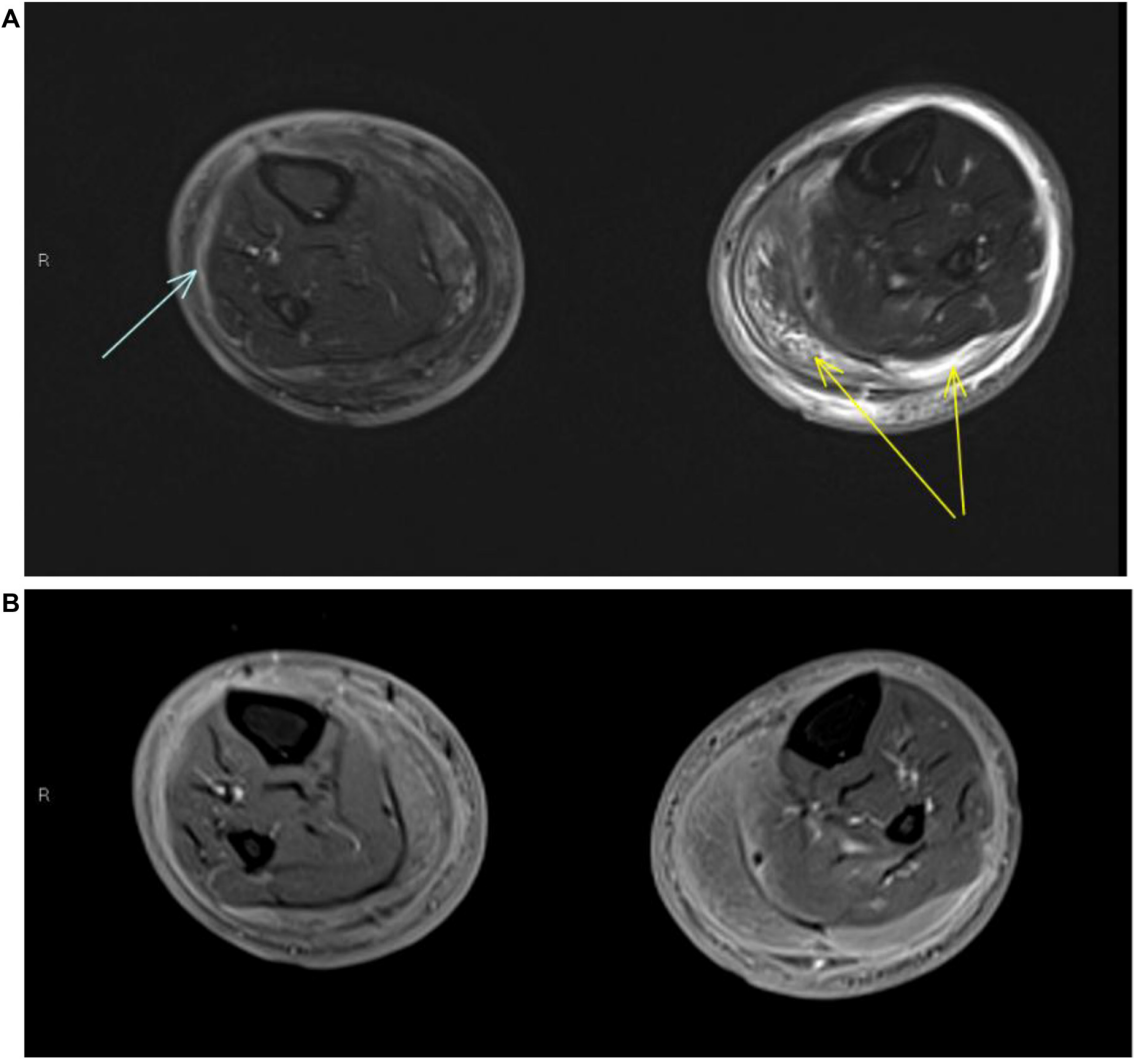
MRI imaging of a 29-year-old male patient with IgG4-related lower limb fasciitis. Axial STIR MRI (A) and postcontrast (B) images demonstrate circumferential thickening of the deep fascia of both lower legs, in keeping with recurrent fasciitis. Superficial circumferential oedema is seen on the right side while a deeper circumferential layer of subcutaneous oedema is seen on the left. Mild oedema deep to the deep fascia on the right side is suggestive of more acute inflammation (blue arrow). The muscles are largely spared, with localised myositis seen in the left medial and lateral gastrocnemius (yellow arrows). (The upper legs were not imaged due to patient intolerance.) MRI, magnetic resonance imaging; STIR, Short Tau Inversion Recovery; IgG4, immunoglobulin G4.
